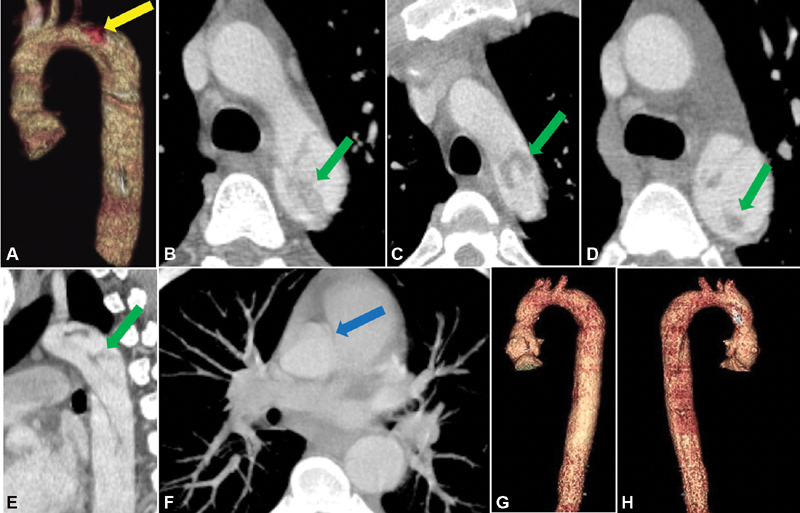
Preoperative computed tomography (CT) images: (
